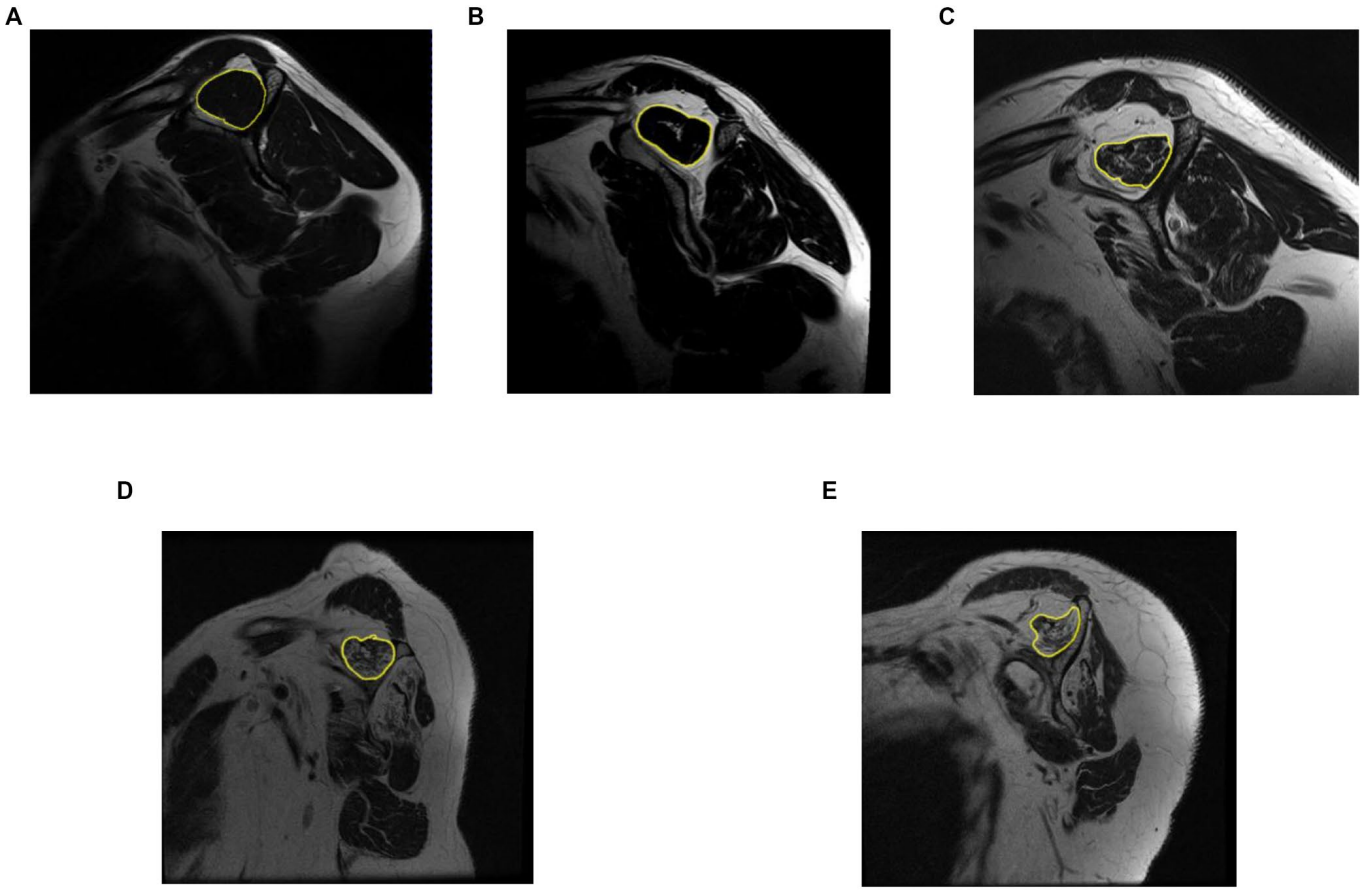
Representative MRI for each Goutallier’s fatty infiltration scale. Level 0,1, 2, 3, and 4, are shown in sub-image (A–E), respectively.
